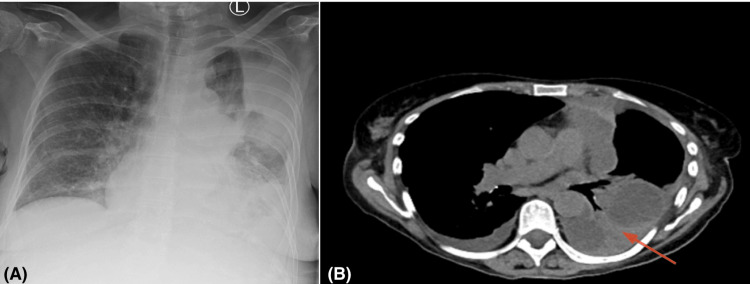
Chest radiograph and CT showing recurrent complex left pleural effusion despite prior video-assisted thoracoscopic surgery (VATS) drainage (A) Chest radiograph (PA) demonstrating near-complete opacification of the left hemithorax with a fluid meniscus and volume loss, compatible with a large recurrent pleural effusion. (B) Axial contrast-enhanced CT chest showing a complex, loculated left pleural effusion (red arrows) with compressed residual lung anteriorly.
